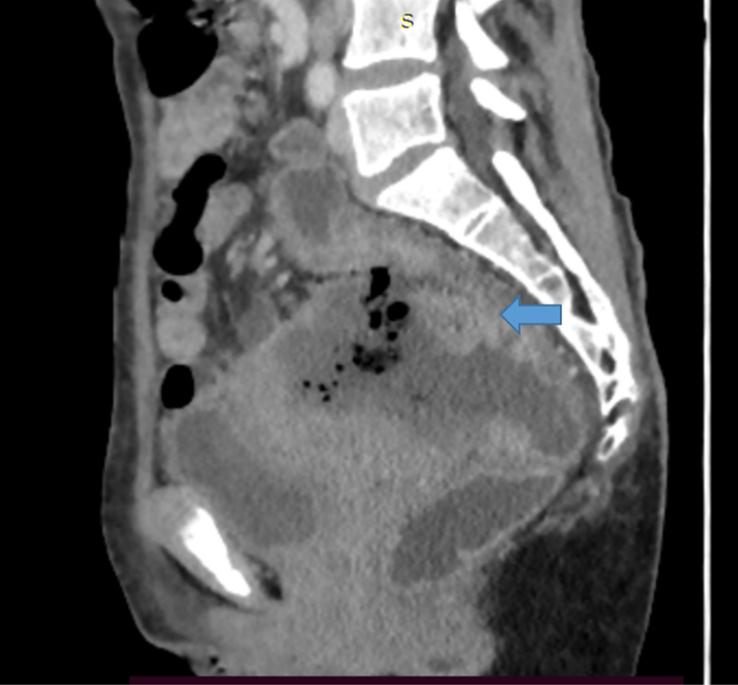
Sagittal section of contrast-enhanced computed tomography (CECT) of abdomen and pelvis showing heterogeneously enhancing asymmetrical circumferential thickening of the proximal rectum and rectosigmoid junction (blue bold arrow).
